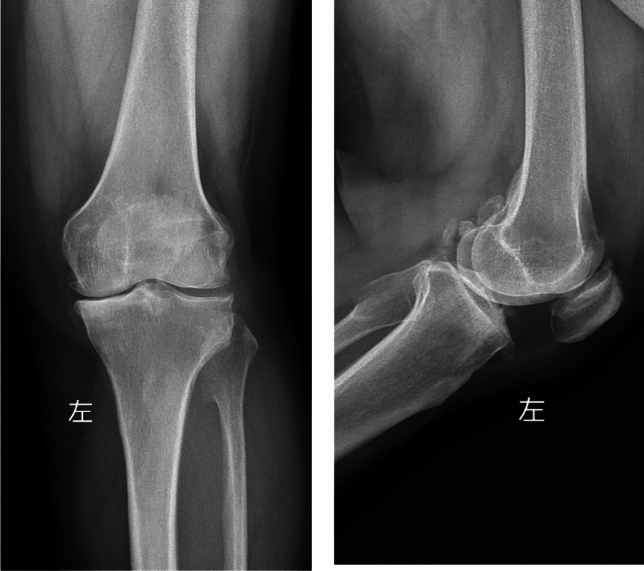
Anterior and lateral X-ray films of the knee joint before operation showing changes in osteoarthritis of the left knee joint, especially in the medial compartment
